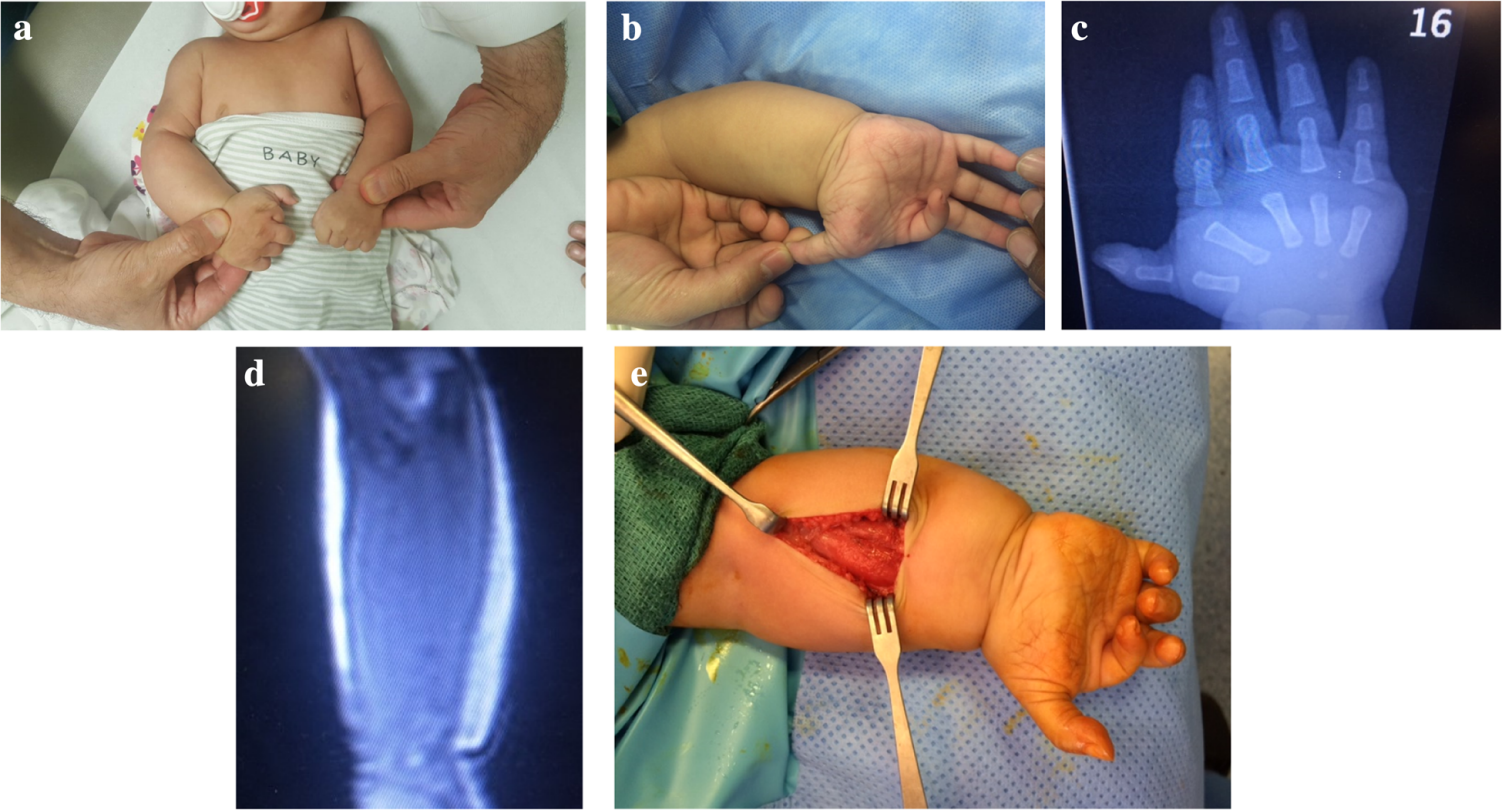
A 2-month-old boy with upper limb MO-hypoplasia IF syndrome. a A view to compare the affected right upper limb to the unaffected left upper limb. Note that the overgrowth is more prominent in the hand/forearm than the arm. b A view of the hand showing hypoplasia and ulnar-deviation of the index finger. Note the wide palm with abnormal palmar creases as well as the hyperextension of the thumb. c Plain X-ray of the hand showing a short hypoplastic index finger and widening of the space between the second and the third metacarpal. d MRI of the forearm showing the “pure” muscle overgrowth. e A view during the muscle biopsy procedure. Note the bulky muscles with a normal subcutaneous fat layer
